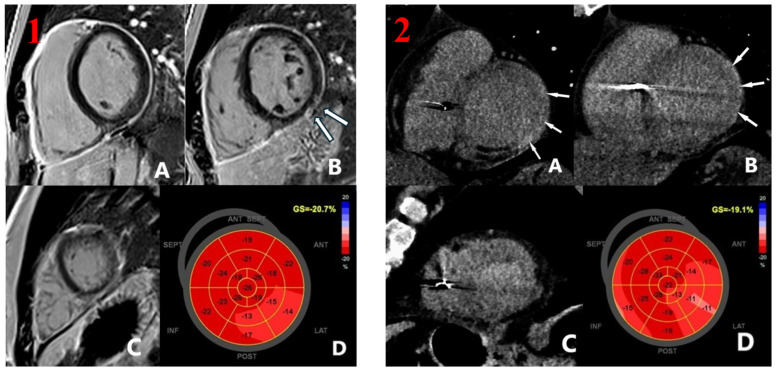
(1) Cardiac Magnetic Resonance: short-axis PSIR (Phase-Sensitive Inversion Recovery) sequences, depicting basal (A), mid-ventricular (B), and apical levels (C), with late gadolinium enhancement observed in the medium inferolateral and inferior walls (B), corresponding to a reduction in regional strain (D). (2) Cardiac Computed tomography: post-contrast sequences showing basal (A), mid-ventricular (B), and apical levels (C), with late iodine enhancement observed in the basal and medium lateral and inferolateral walls (B), corresponding to a reduction in regional strain (D).
